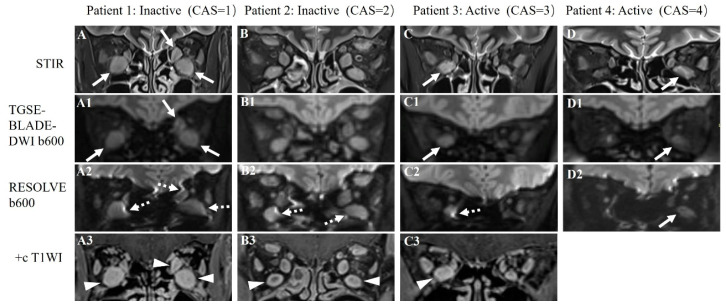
Representative STIR (A–D), TGSE-BLADE-DWI b600 (A1–D1), and RESOLVE b600 images (A2–D2) of four TAO patients with different clinical activity scores (CAS). Patient 1 was a 43-year-old male TAO patient with CAS = 1, with bilateral enlarged inferior and left medial rectus muscles shown on the STIR sequence (A) that could be clearly displayed on TGSE-BLADE-DWI (white arrows in A1); slight to moderate susceptibility artifacts and geometric distortions were seen with RESOLVE (dotted arrows in A2). Patient 2 was a 45-year-old male TAO patient with CAS = 2; the bilateral superior, inferior, and medial rectus muscles were enlarged in size and were clearly visualized without artifacts on TGSE-BLADE-DWI (B1); the bilateral inferior rectus muscles were slightly distorted on RESOLVE (dotted arrows in B2). Patient 3 was a 42-year-old female TAO patient with CAS = 3; the right inferior rectus was enlarged on STIR (C) and is clearly shown on TGSE-BLADE-DWI (white arrow in C1) but was severely affected by susceptibility artifacts and geometric distortions on RESOLVE (dotted arrow in C2). Patient 4 was a 55-year-old female TAO patient with CAS = 4; the left inferior rectus was enlarged on STIR and was displayed well both in TGSE-BLADE-DWI and RESOLVE (white arrows in D1, D2). Contrast-enhanced T1WI (+c T1WI) scans (A3–C3) were performed in patient 1–3, and the coronal images identified the enlargement of multiple EOMs (arrowheads in A3, B3 and C3).
